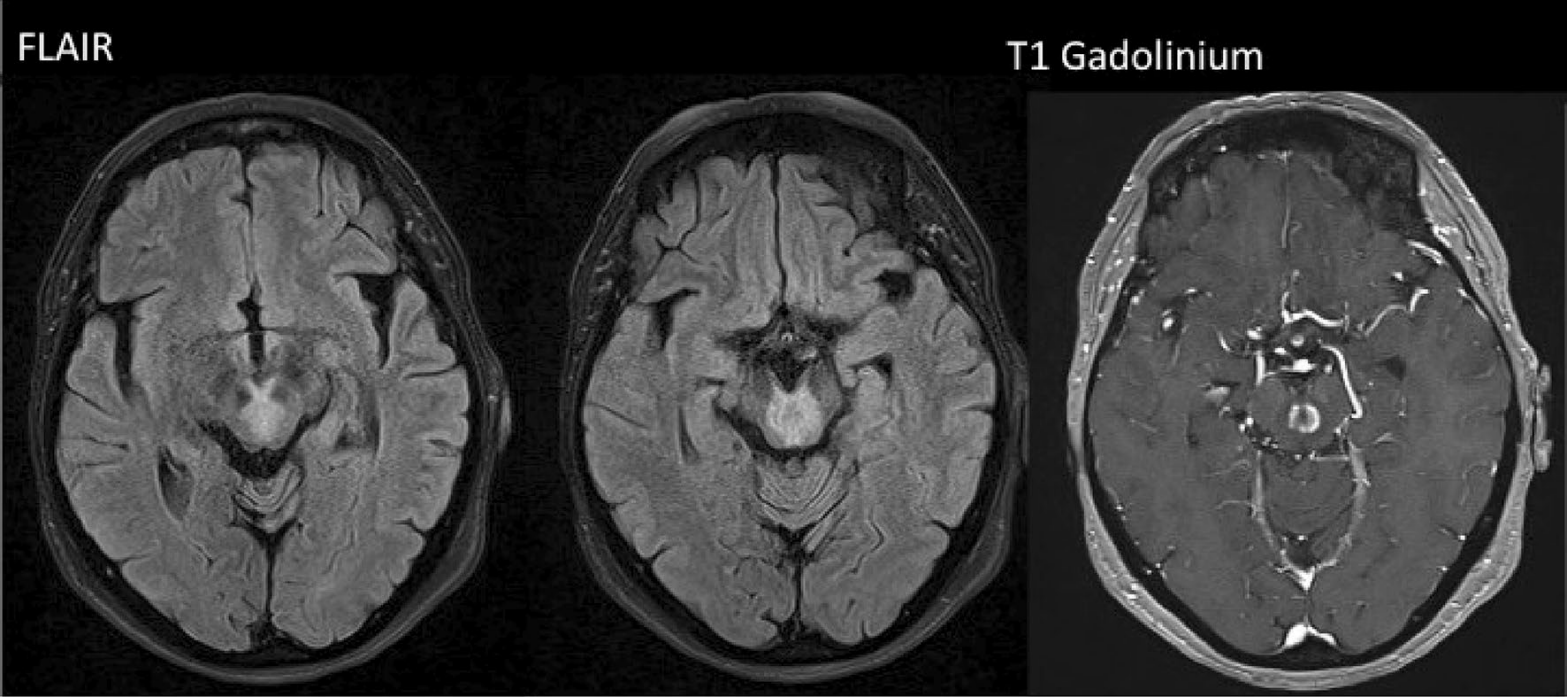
Patient 1 (N1) brain MRI. Note the central midbrain lesion with contrast enhancement
